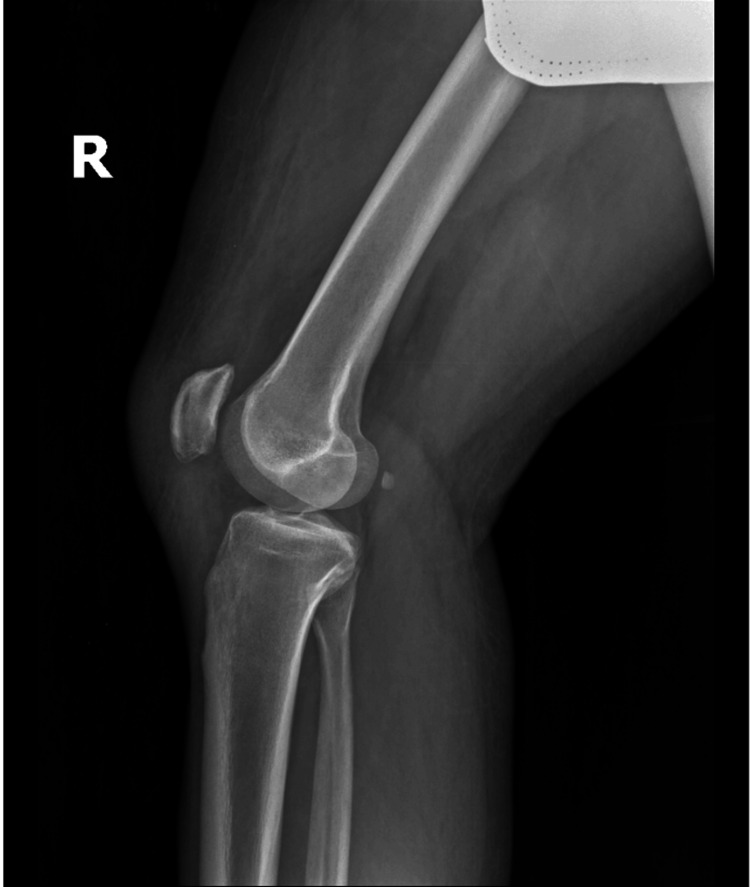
Postoperative lateral radiograph of the right knee Lateral radiograph of the right knee obtained after surgical intervention demonstrates restored patella height and alignment with no hardware or bony abnormalities noted.
